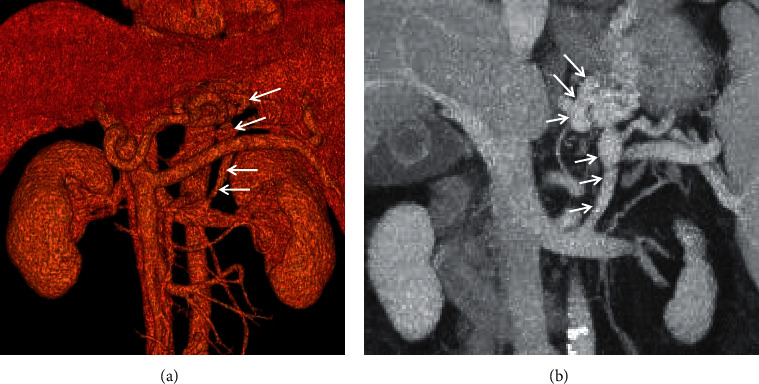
Gastrorenal shunt. A 62-year-old male with a four-year history of unexplained liver cirrhosis presented with recurrent hematemesis. The contrast-enhanced CT scan on the portal vein phase demonstrated that gastrorenal shunt (white arrow) manifested as enhancement of abnormally dilated vessels originating from the gastric vein to the left renal vein. (a) Three-dimensional reconstruction; (b) coronal contrast-enhanced CT.
